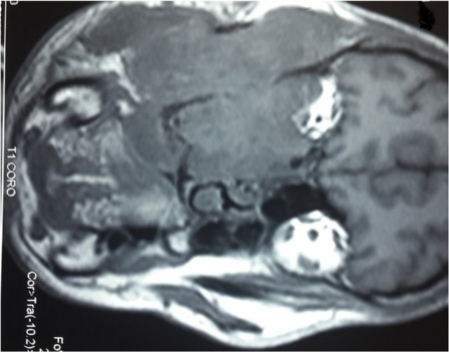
Coronal T1-weighted magnetic resonance image of the facial area showing an invasive tumor occupying her right infratemporal fossa and maxillary sinus with irregular boundaries, heterogeneous intermediary signal at T1 weight, with sphenoidal, ethmoidonasal, nasopharyngeal extension
